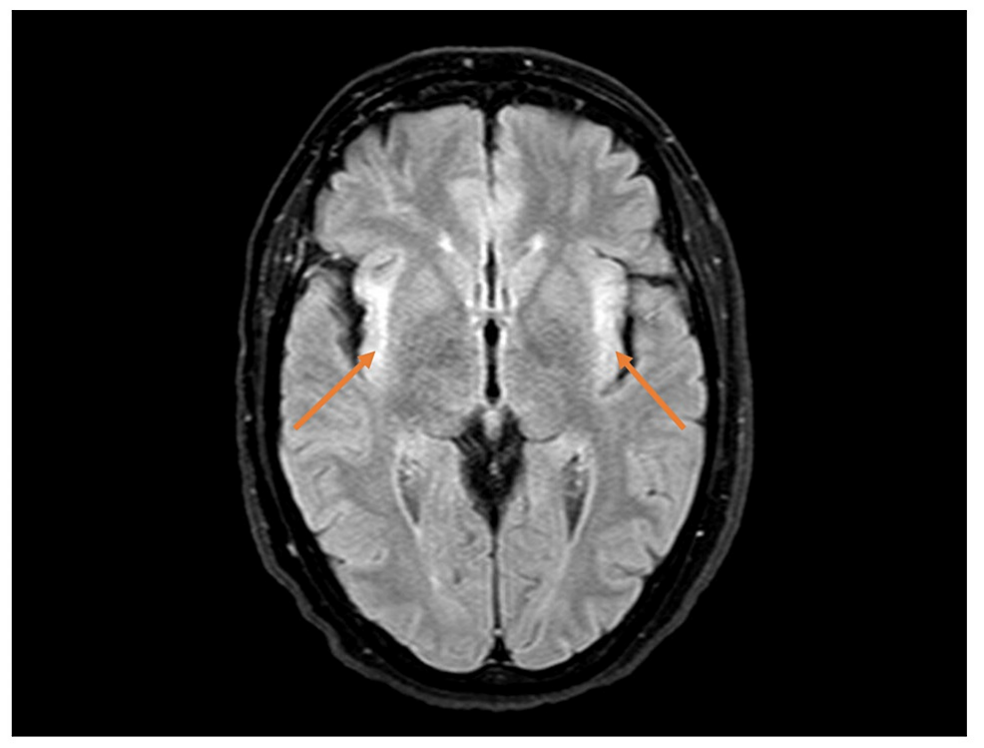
Patient's brain MRI Axial T2 flair showing bilateral hyperintensity of the insular cortices
